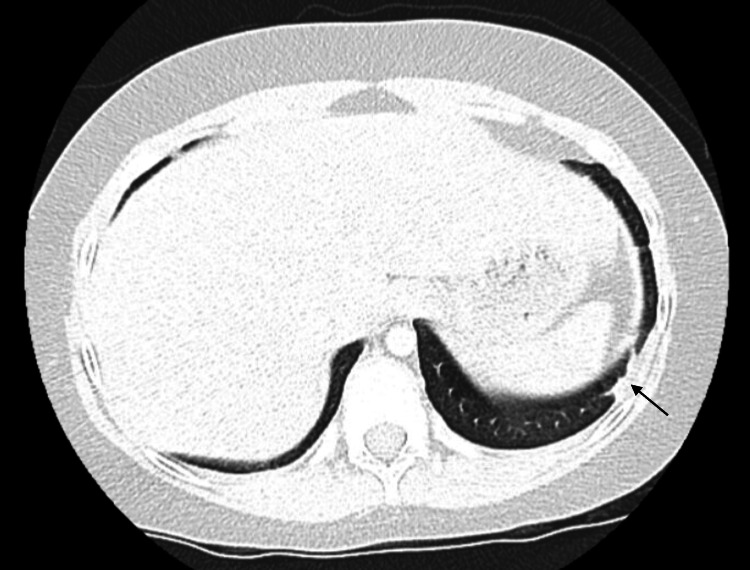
CT scan of the chest showing a subpleural nodule.
